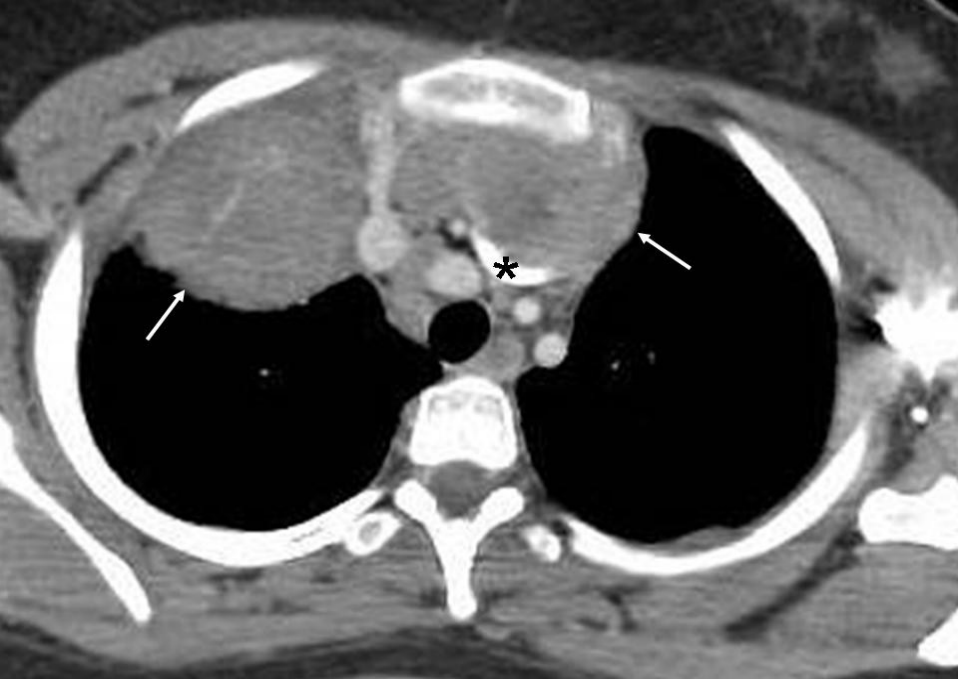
Contrast enhanced CT of the chest obtained in a patient with Hodgkin lymphoma shows a confluent mass (arrows) in the mediastinum anteriorly. The mass indents the superior aspect of the left brachiocephalic vein (asterisk). Small amount of pleural effusion is seen on the left side. It is to be noted that a mass originating in the mediastinum forms an obtuse angle with the adjacent lung, often has a smooth contour and can extend across the midline involving both hemithoraces. On the other hand, an intra-pulmonary mass forms an acute angle with the adjacent lung, does not extend across the midline, and may contain an air-bronchogram
